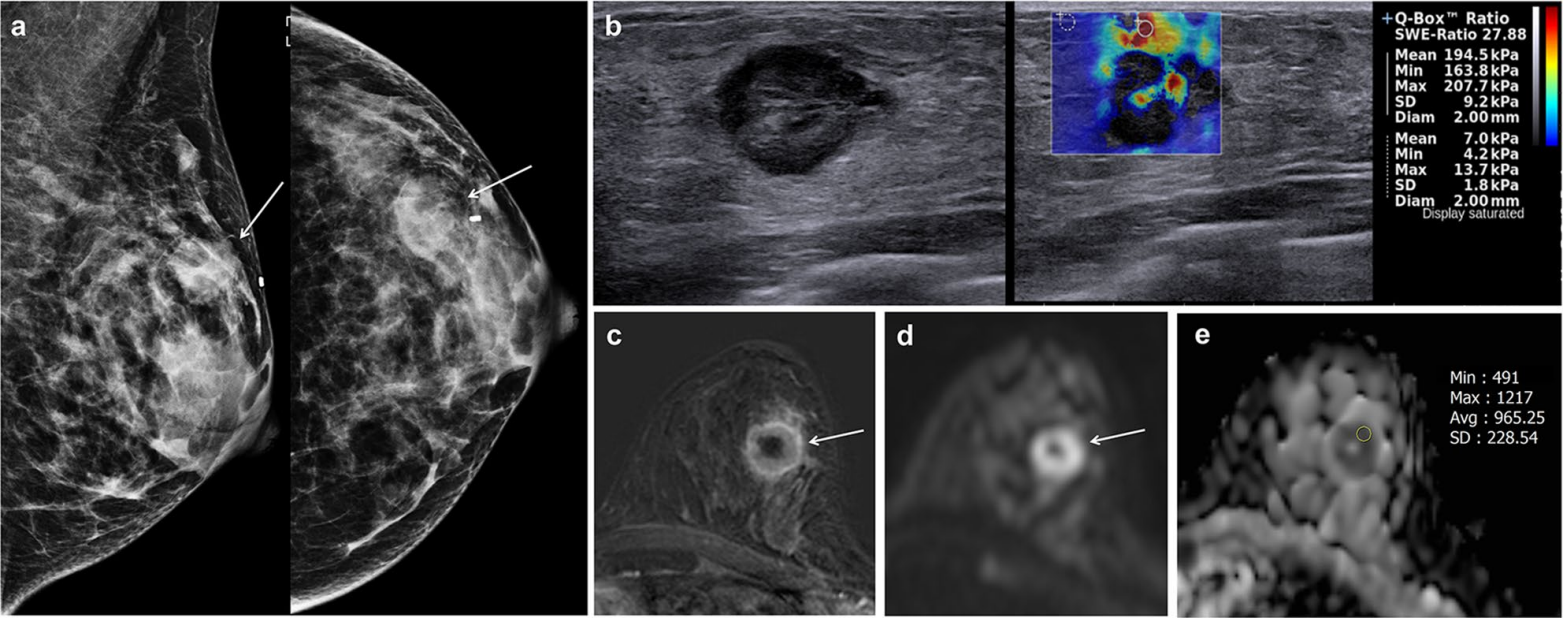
Imaging findings of triple-negative invasive breast cancer of no special type in the left breast of a 67-year-old woman. (a) Images obtained with mammography in the left mediolateral oblique (left) and left craniocaudal view (right) shows an irregular hyperdense mass (arrows) in the upper outer breast. (b) B-mode ultrasound image (left) shows a 22-mm microlobulated heterogenous mass with posterior enhancement. Shear-wave elastography (SWE, right) values are measured, with a mean elasticity of 194.5 kPa and a maximum elasticity of 207.7 kPa. (c) Axial contrast-enhanced T1-weighted subtraction magnetic resonance imaging (MRI) shows a round mass with rim enhancement (arrow). The mass shows early rapid and delayed washout enhancement. (d) Axial diffusion-weighted MRI (b value, 1000 s/mm^2^) demonstrates a mass with high signal intensity (arrow). (e) On the reconstructed apparent diffusion coefficient (ADC) map, the ADC value of the mass is 0.965 × 10^−3^ cm^2^/s. US-guided core-needle biopsy revealed invasive breast cancer of no special type that was estrogen receptor negative, progesterone receptor negative, and human epidermal growth factor receptor 2 negative.
